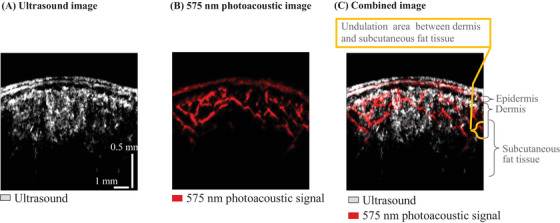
Typical image of photoacoustic measurement results combining ultrasound signals. (A) Ultrasound image. (B) 575‐nm photoacoustic image. (C) Combined image. In the ultrasound signal, findings with undulation structures are shown in the depth region indicated by the yellow line.
